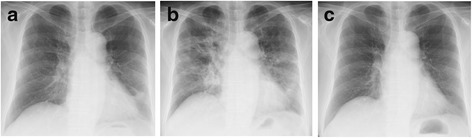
Chest X-ray images. a Chest X-ray on admission. b Chest X-ray at the time of worsening of respiratory failure. c Chest X-ray after steroid treatment
